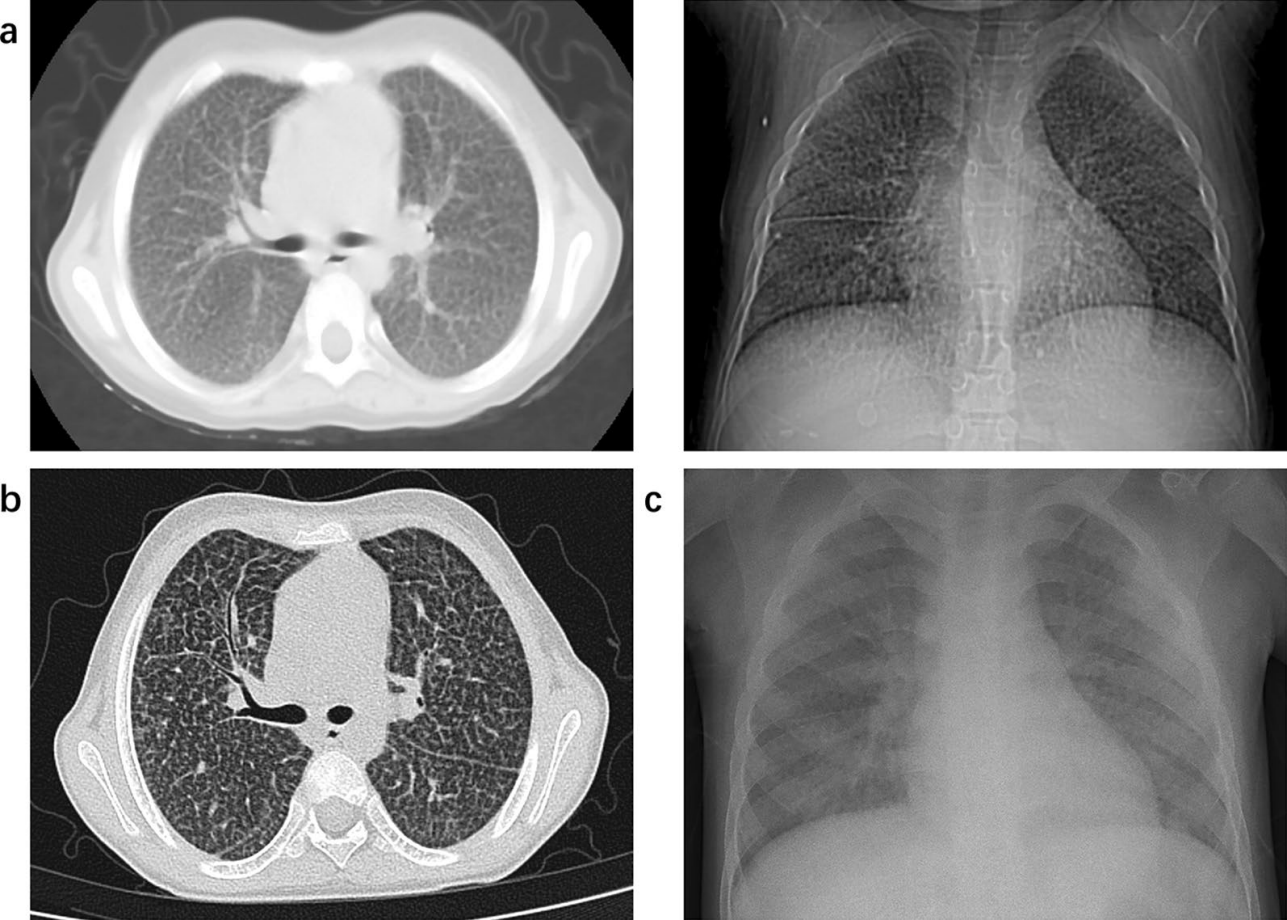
The chest radiography of Patient A. (a) post-LT: typical imaging presentation of Niemann-Pick Disease; (b) in the early stage (the 2nd day) of SARS-CoV-2 infection: no visible change; (c) in the later stage (the 21st day) of SARS-CoV-2 infection: increased and blurred texture in both lungs with patchy exudate
